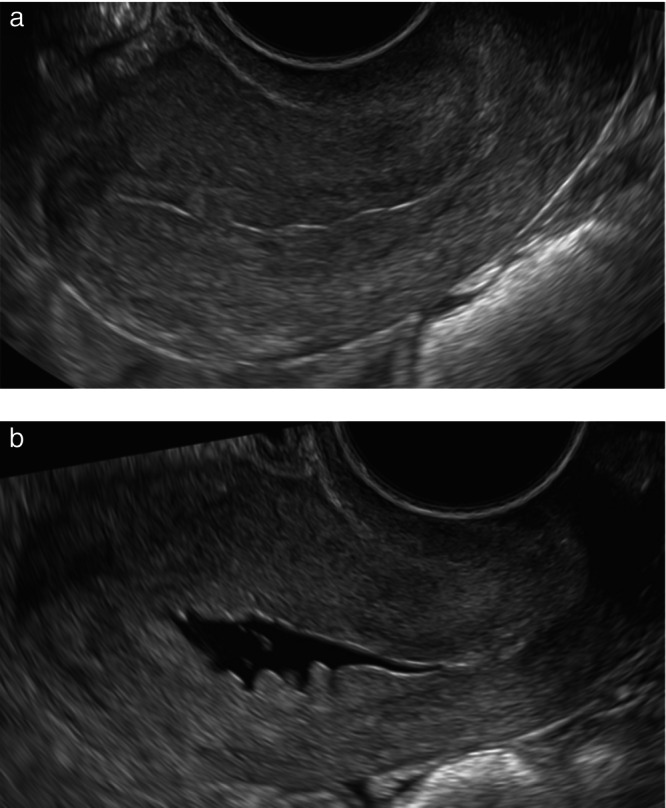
Ultrasonographic examples of a non‐linear endometrial midline, in sagittal section. (a) Without fluid instillation, it can be seen that the endometrial midline is not linear. (b) After fluid instillation, note the irregular and polypoid delineation of the cavity.
